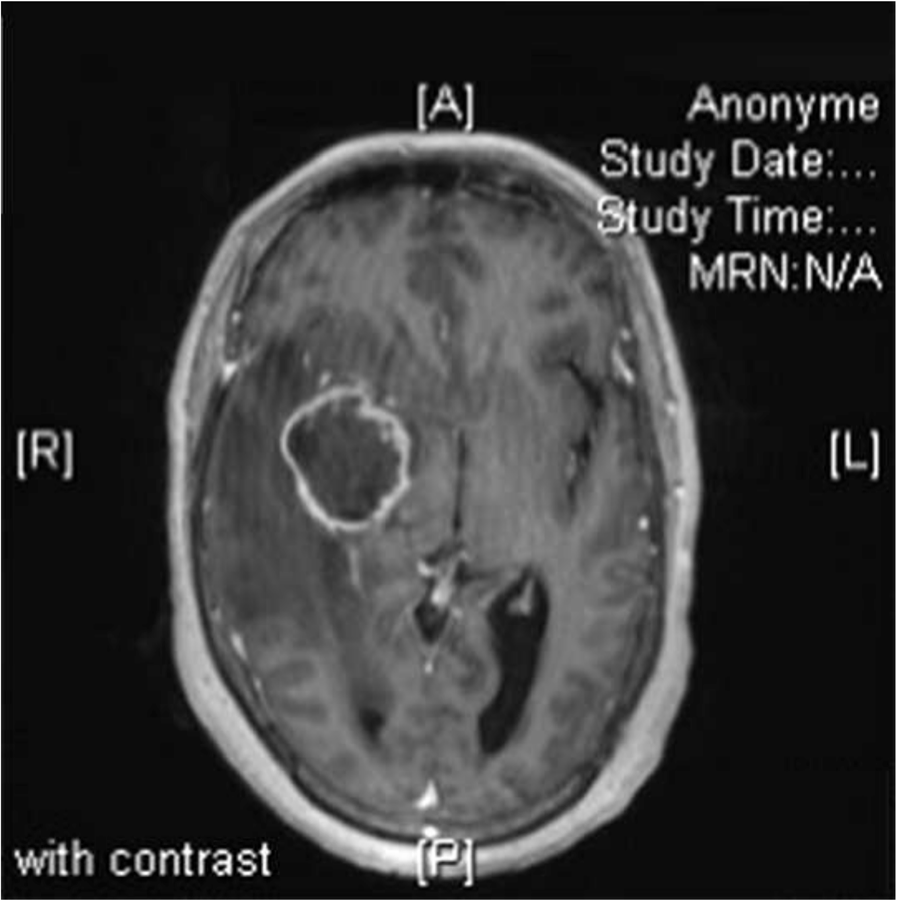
Brain magnetic resonance imaging revealed a single 40×35 mm tumor in the right mesial temporal region and a mass effect compression of the right lateral ventricle with transtentorial herniation
